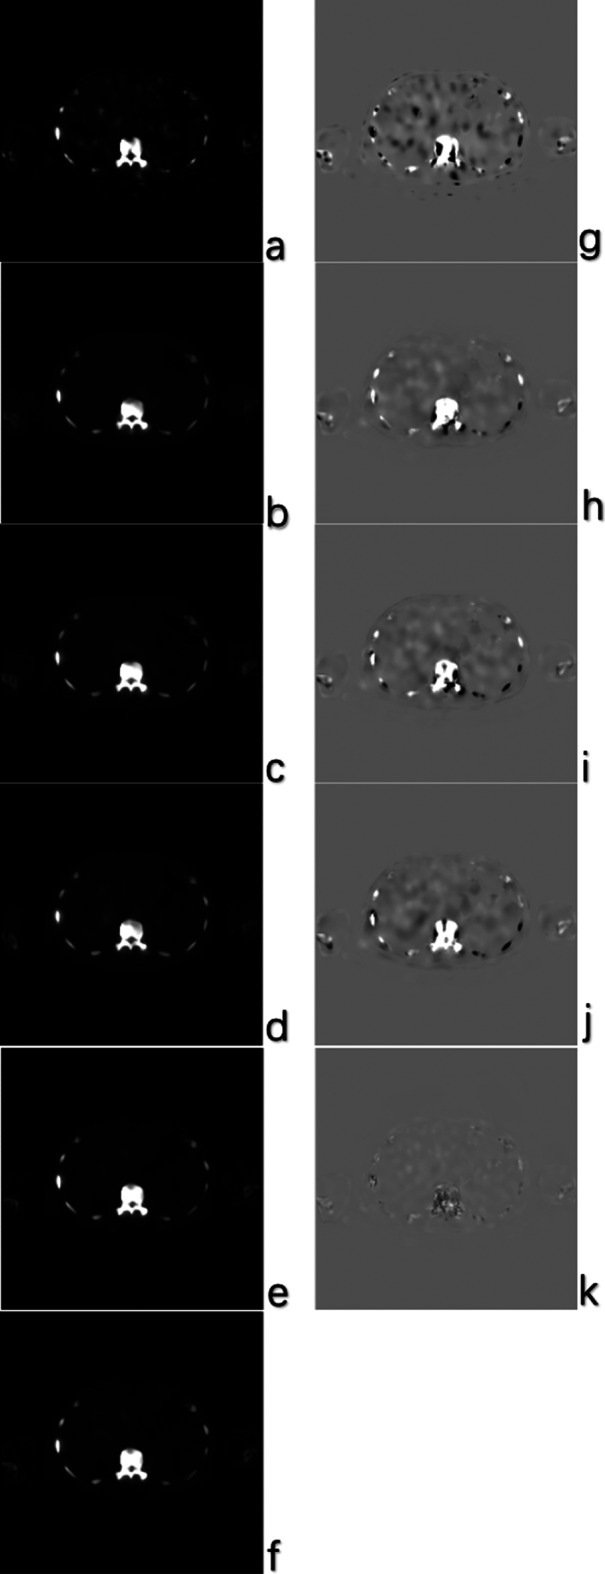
Clinical assessment results of image quality for 1/7 SPECT, proposed method and the standard SPECT. The average scores for the 1/7 SPECT, proposed method and the standard SPECT are 2, 3.85 and 3.85 in terms of general image quality, 1.8,3.8 and 4.15 for details of contrast, 2, 3.75 and 3.9 for presence of artifacts and 1.95, 3.85 and 4.15 for diagnostic confidence. 1/7 SPECT is significantly different from SPECT image enhanced by proposed method under Wilcoxon test (*P* < 0.05) for all evaluation metrics. Compared to the standard SPECT, proposed method achieved the same general image quality (*P* > 0.999), similar detail of 99mTc-MDP (*P* = 0.125), presence of artifacts (*P* = 0.531) and the diagnostic confidence (*P* = 0.1875)
